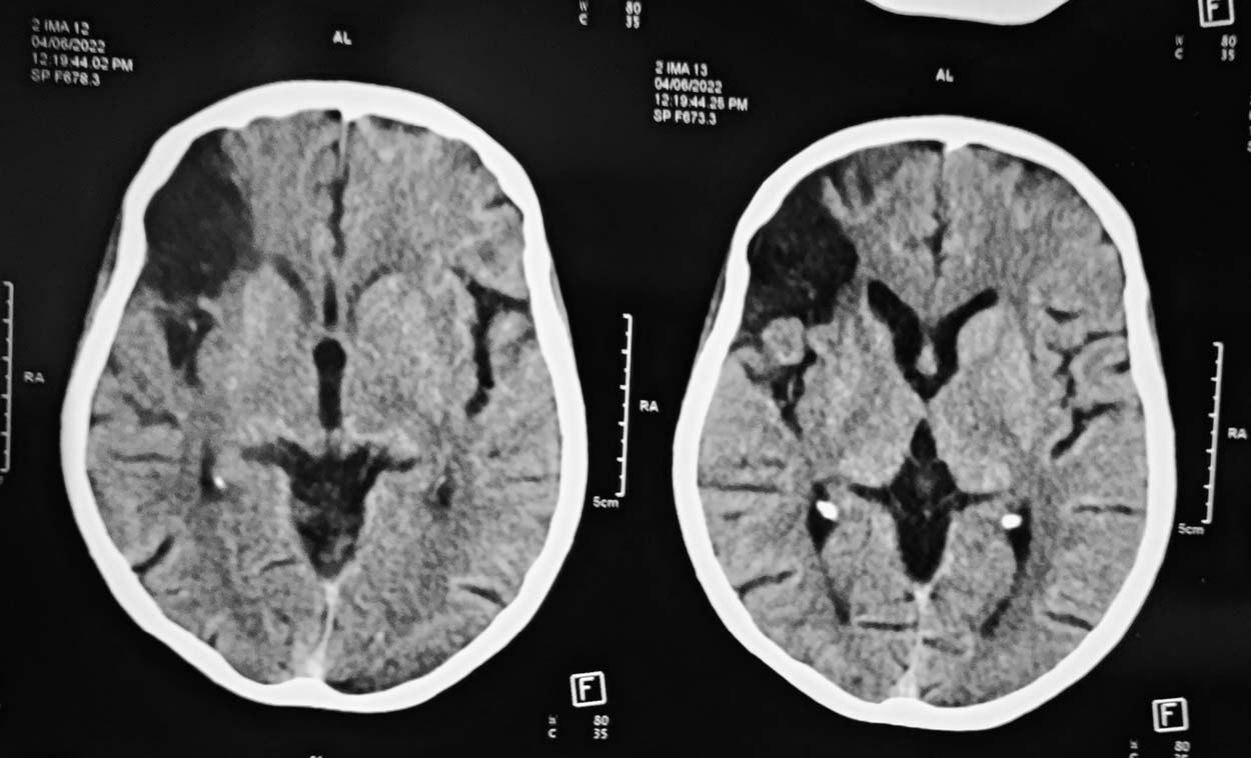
NC-CT scan of head showing encephalomalacic changes in the right anterior frontal lobe region.
